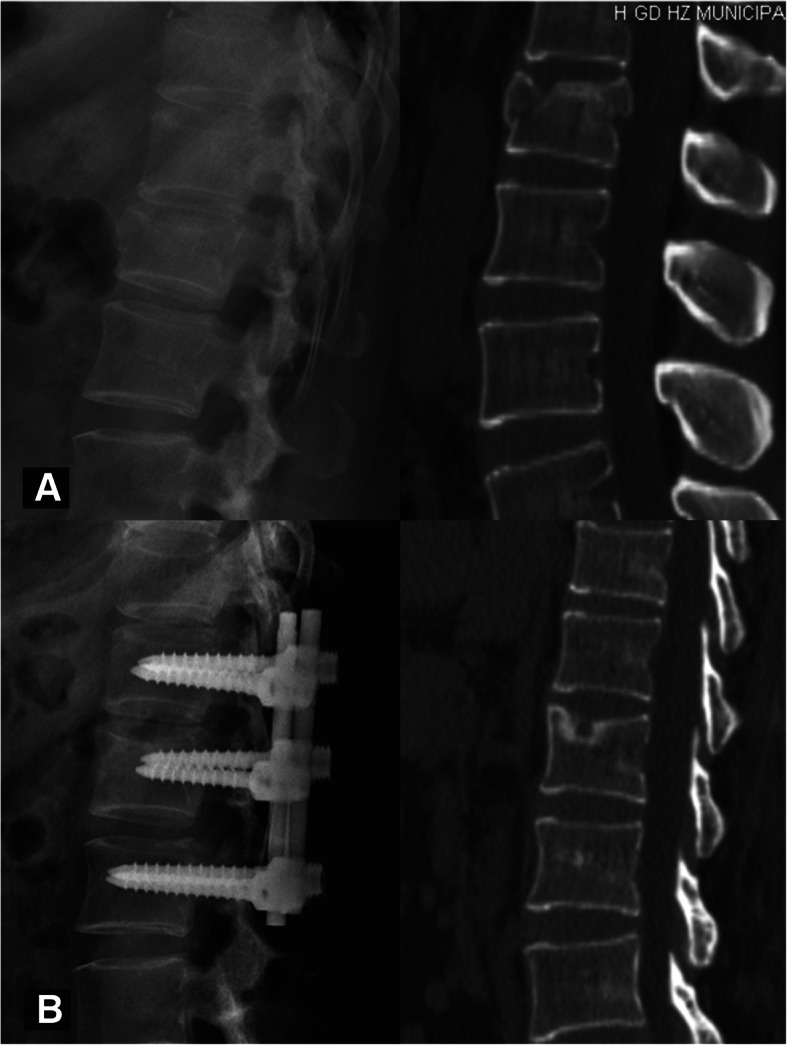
A 48-year-old female had a burst fracture at L1, with an ASIA grade for neurological function of E on admission. a Preoperative X-ray and CT images show an A3 burst fracture. b X-ray and CT images at 14 months after surgery show a defect region smaller than 1/3 of the vertebral body volume with violation of the upper endplate, which was classified as HPC type II
